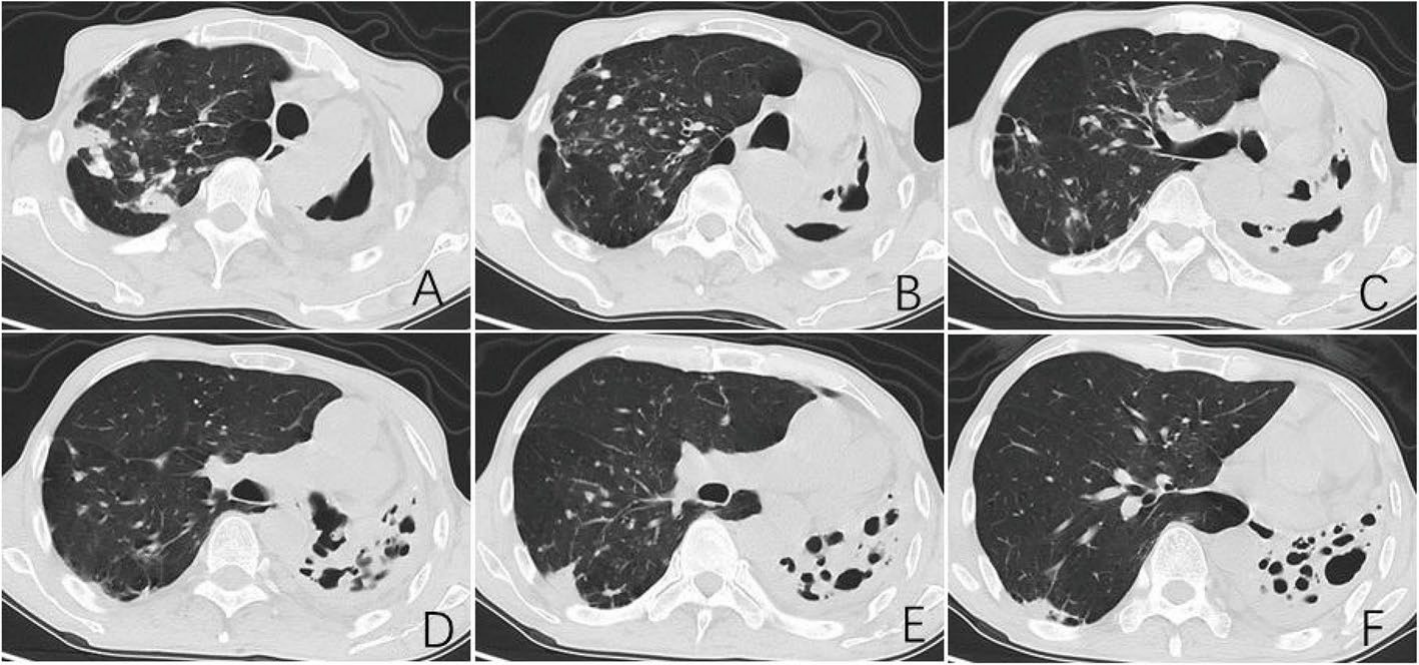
Representative chest CT findings in a 61-year-old male with DR-TB. Key features include: (i) destroyed left lung with segmental consolidation and air bronchograms; (ii) worm-eaten cavities; and (iii) right-sided emphysema
